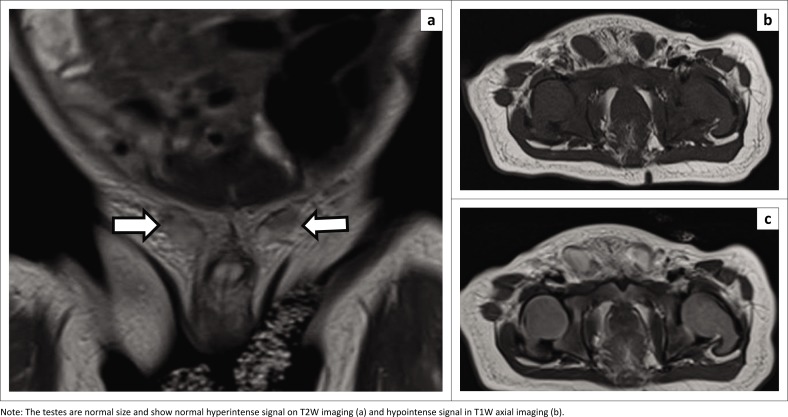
(a) Coronal T2-weighted (T2W), (b) T2W axial and (c) T1-weighted (T1W) axial magnetic resonance images in a 12-month-old male with bilateral undescended testes, demonstrating ectopic testes at the superficial inguinal ring (white arrows).
